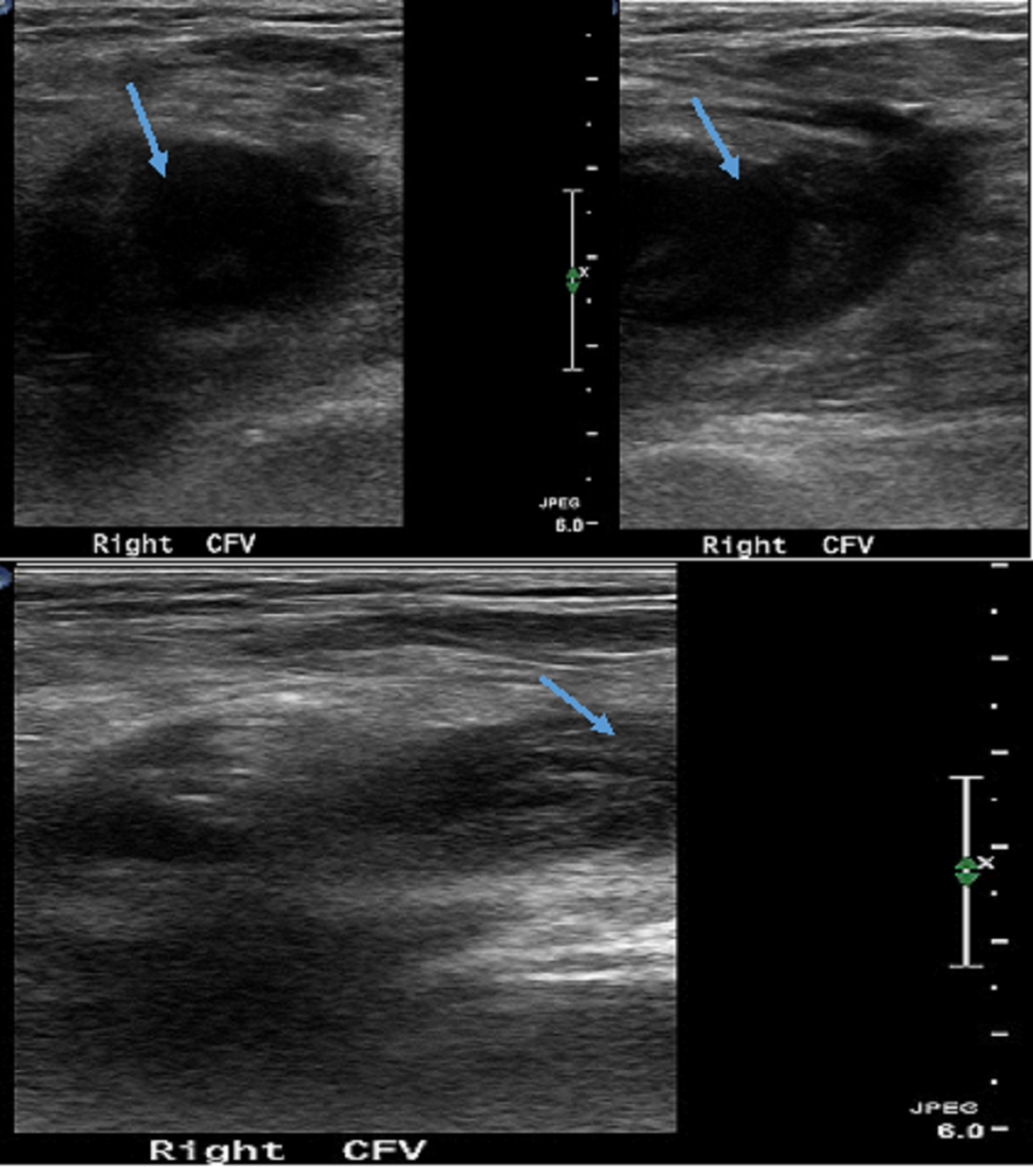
Doppler study showing thrombosis and non-compressible right common femoral vein (arrows)
